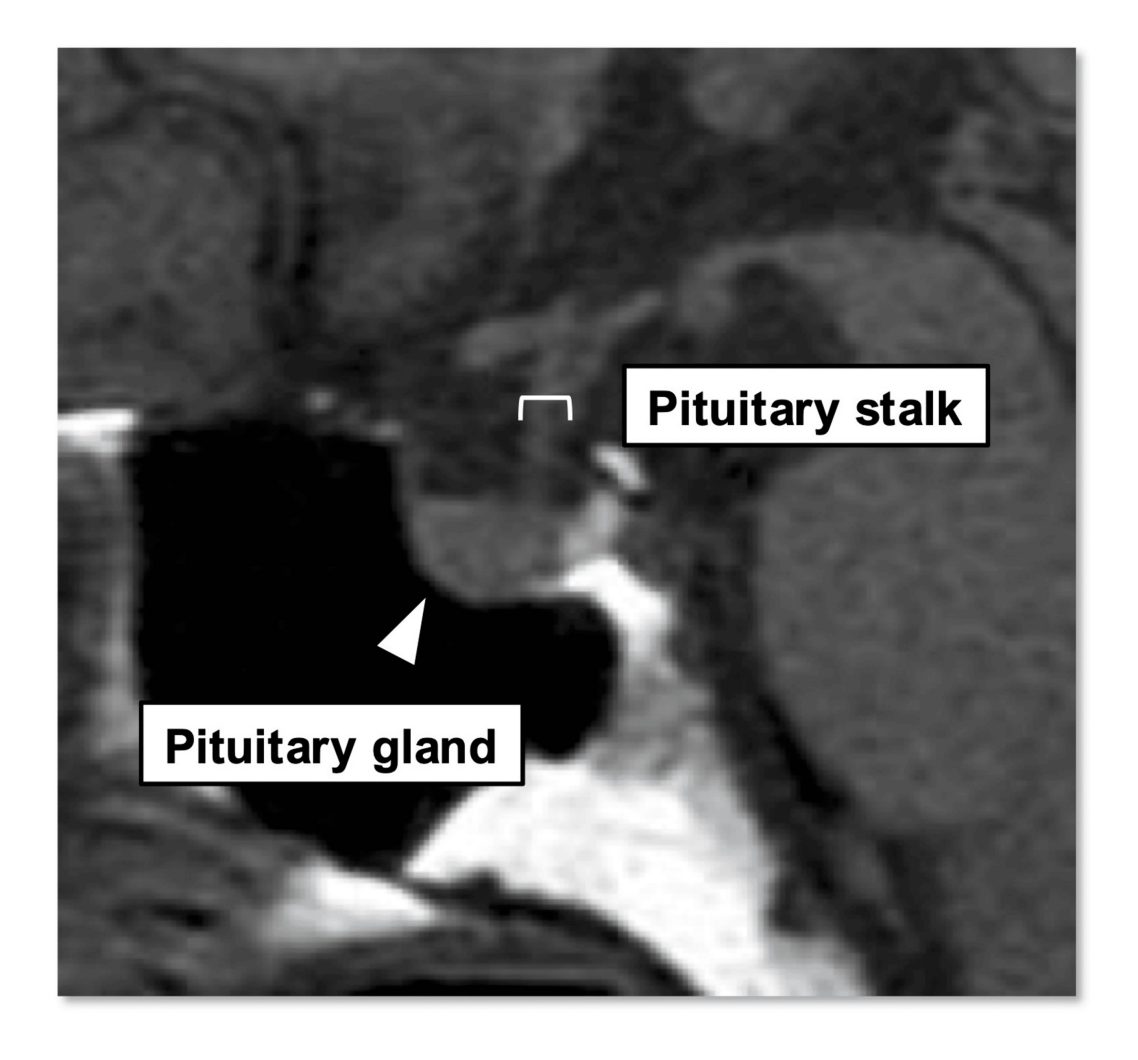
Measurement sites on MRI of the pituitary gland
